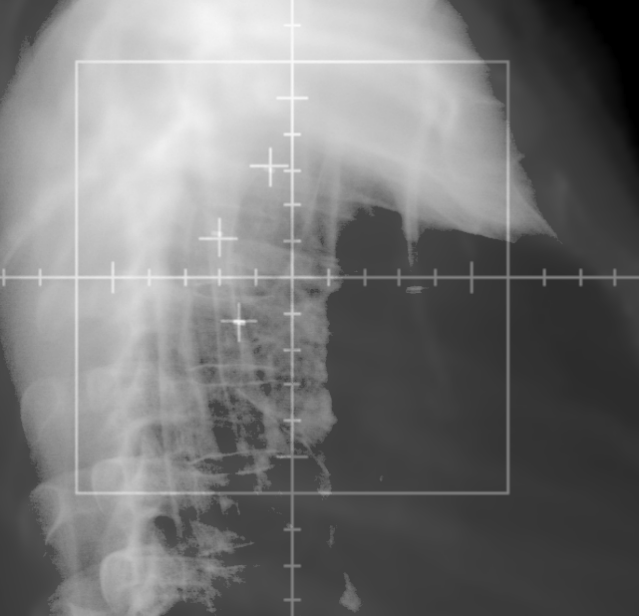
Matching of digital reconstructed radiograph to kilovoltage image (lateral view).
